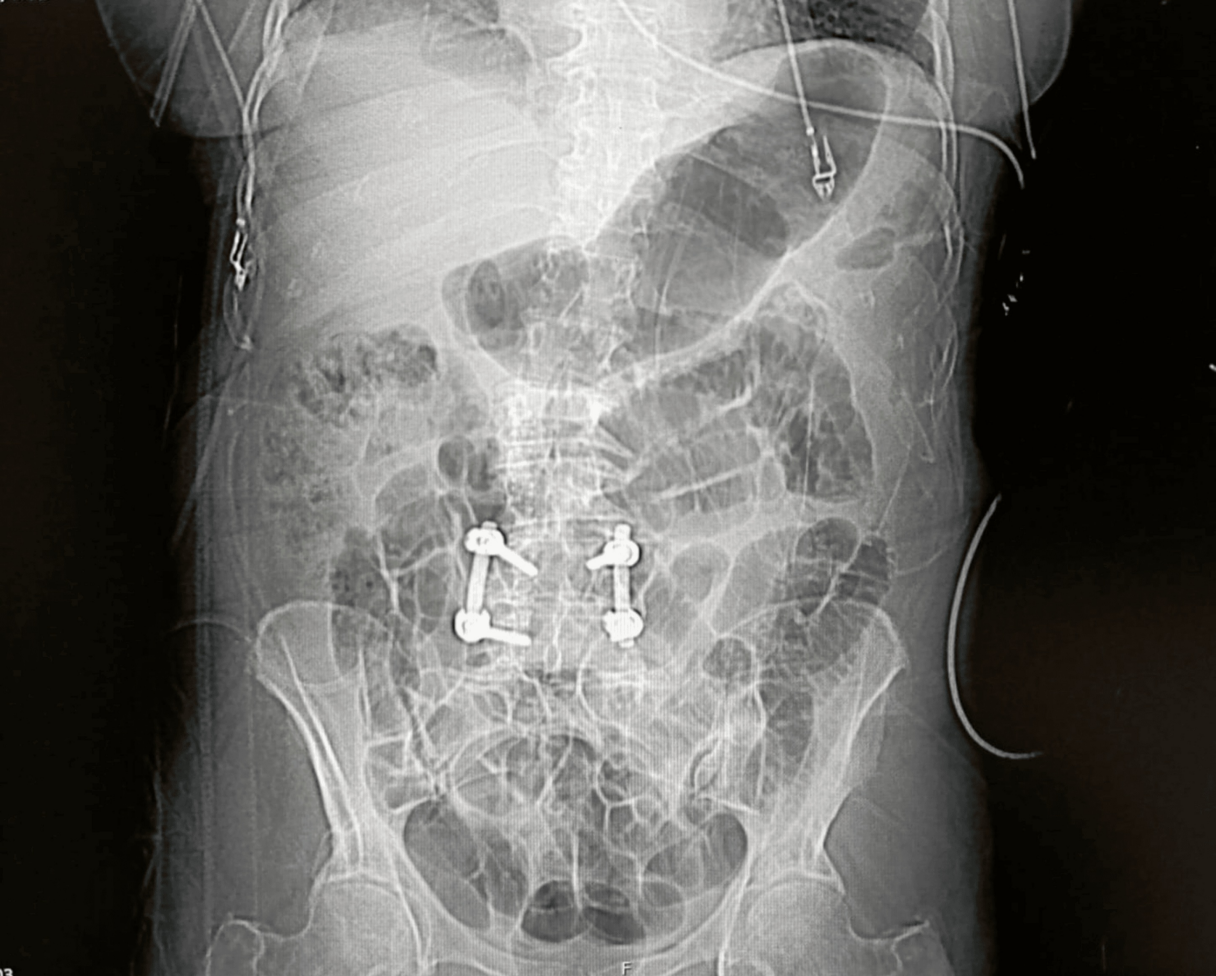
Postoperative spinal CT scan Thoracolumbar coronal CT image showing spinal fusion and instrumentation at L4-L5.
